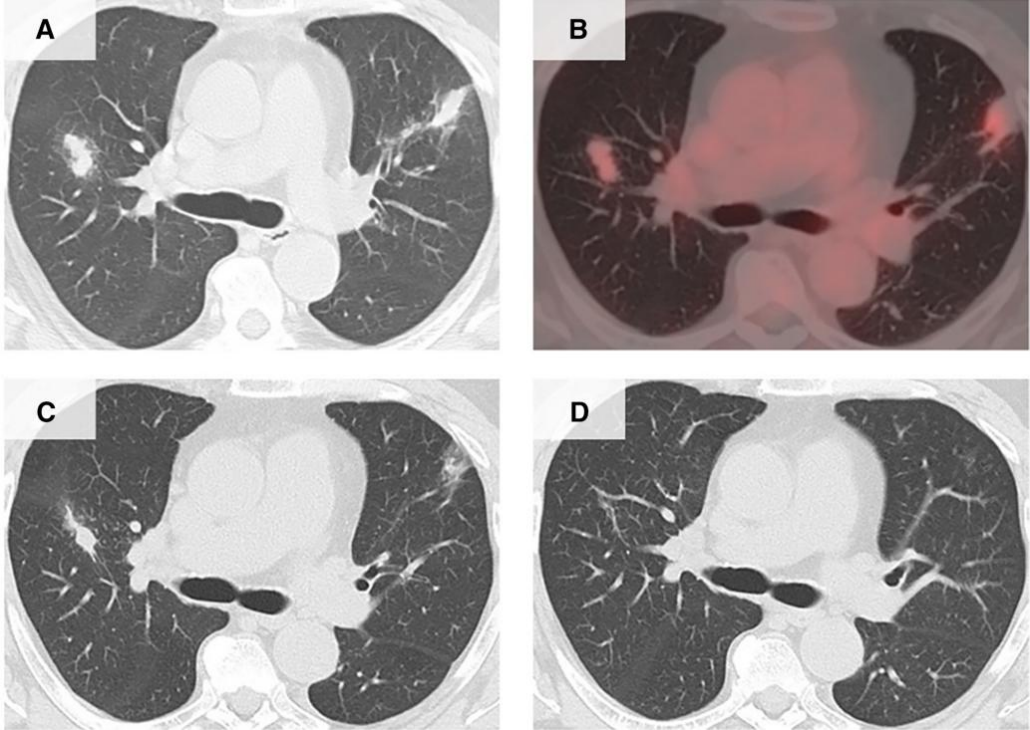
(A) Initial chest computed tomography (CT) showing ground-glass opacities in both lungs. (B) Positron emission tomography scan showing fluorodeoxyglucose uptake with a maximum standardized uptake value of 3.78 in the left lung lesion and 4.29 in the right lung lesion. (C) Follow-up CT after 2 months of corticosteroid therapy showing partial regression. (D) Follow-up CT 4 months after praziquantel treatment revealing near-complete resolution of the lesions.
